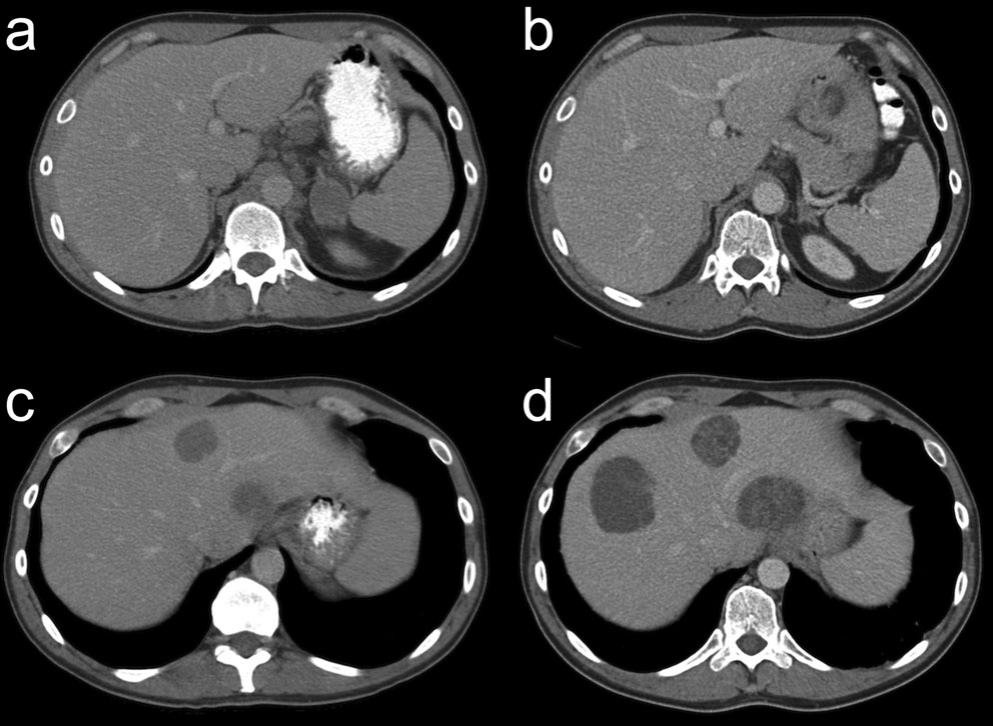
Contrast-enhanced axial CT images before (a, c) and after (b, d) the first line of chemotherapy showed a regression of the left adrenal metastases (a, b) and a clear progression of the liver metastases (c, d)
